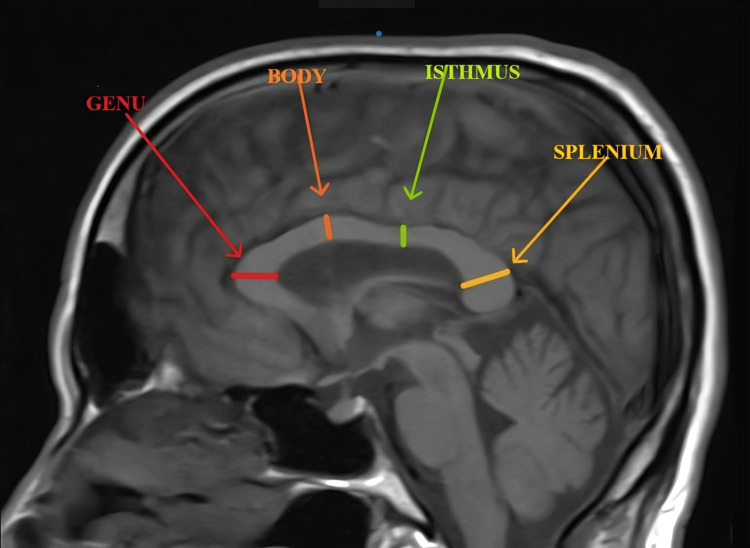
Mid-sagittal T1-weighted image showing the measurement of the maximum thickness of various parts of the corpus callosum
